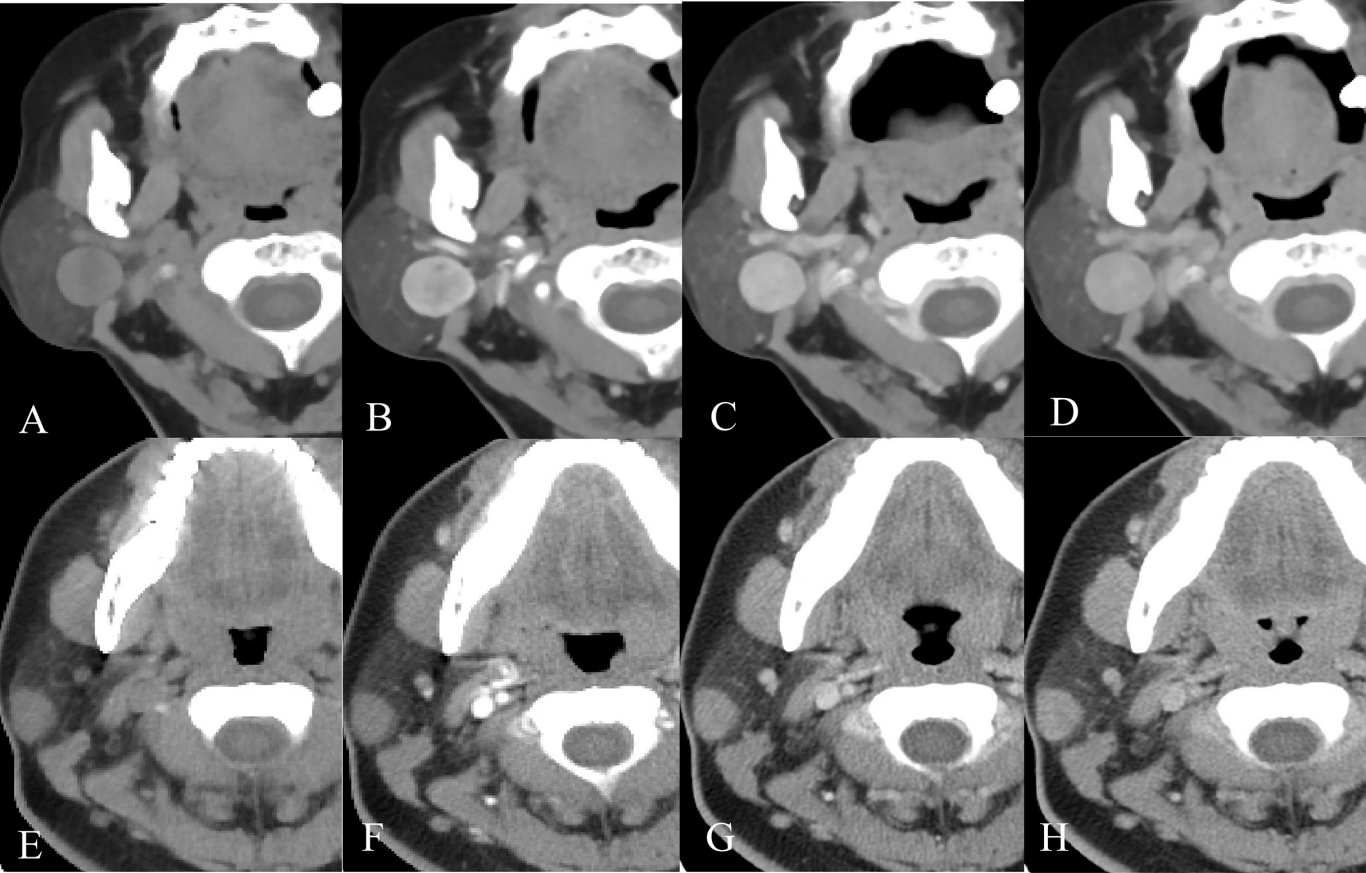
Example images of four-phase CT scans of PA and BCA. (A-D) Axial CT images of a 68-year-old female patient with a right-sided painless neck mass in the plain, arterial, venous, and delayed phases, separately. After CT examination, surgical pathology confirmed that the mass was BCA. (E-H) Axial CT images of a 23-year-old female patient with a right-sided painless neck mass in the order of plain, arterial, venous, and delayed phases. It was confirmed as PA by surgical pathology.
